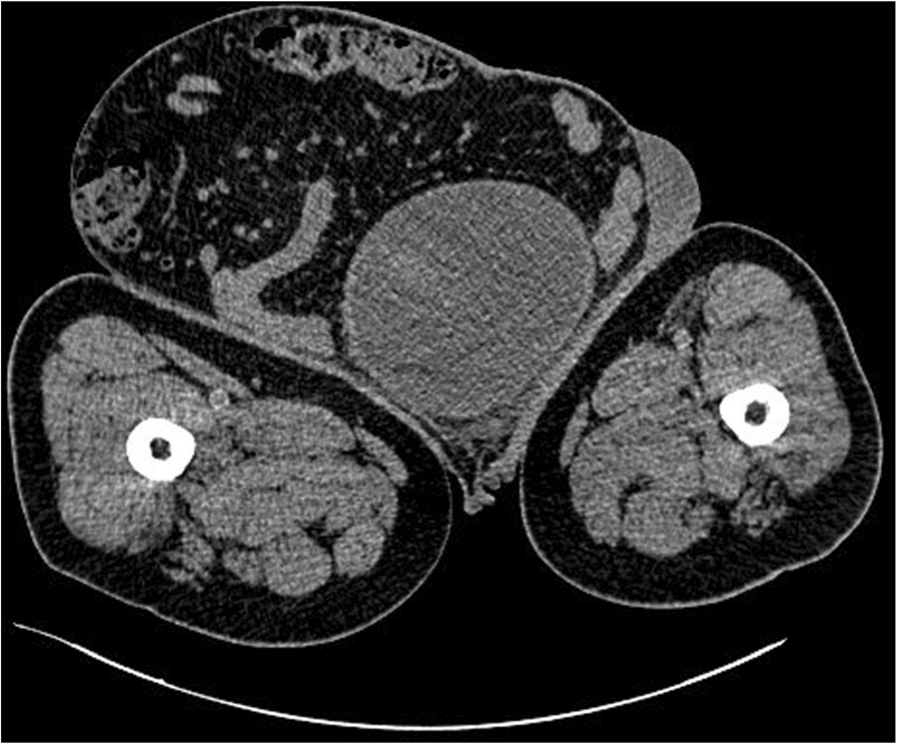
Axial computed tomography demonstrating urinary bladder and bowel loops at the level of the femur
